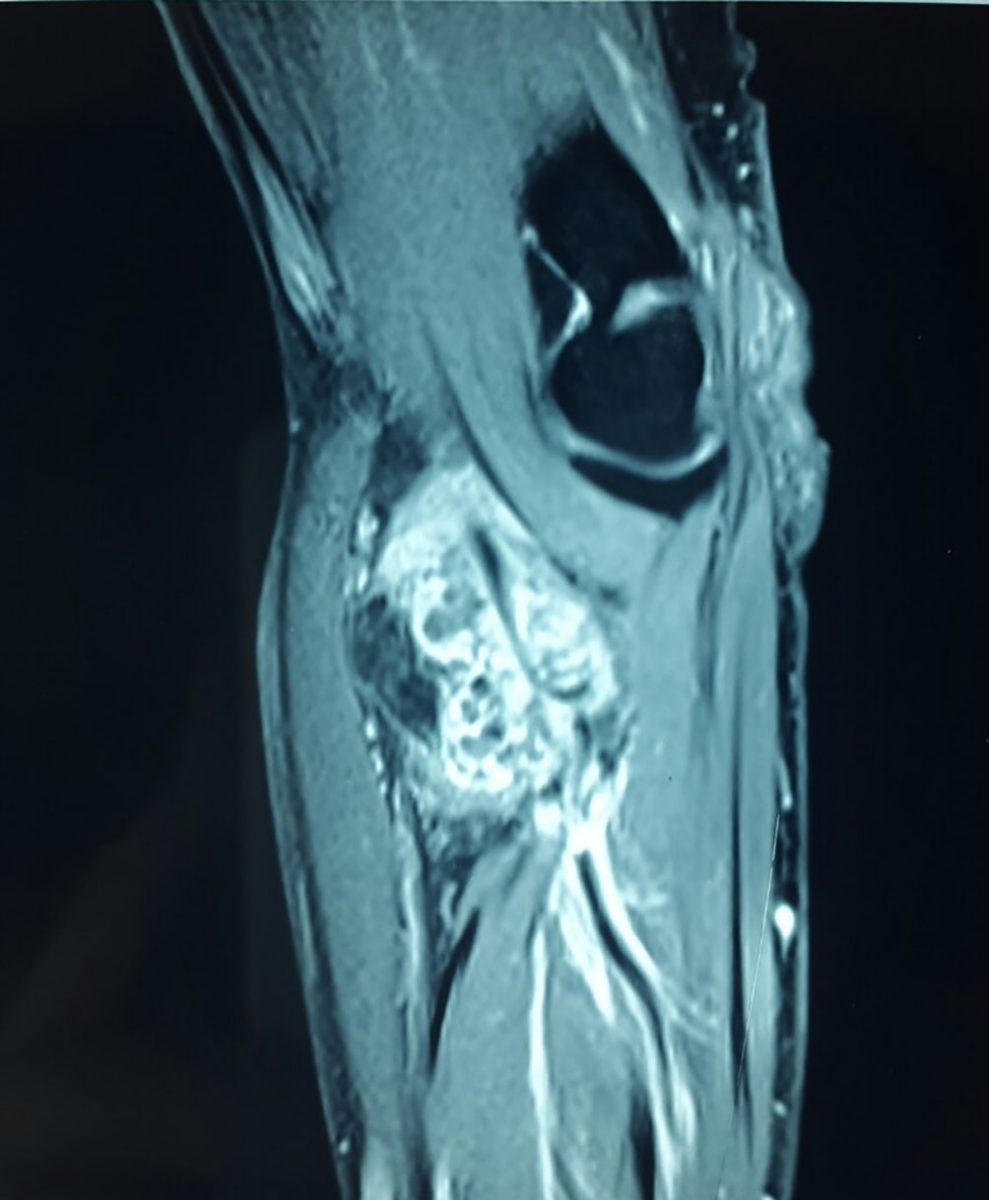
Sagittal magnetic resonance imaging (MRI) image of the left front arm with a lobulated complex mass with frond-like appearance extending from the bicipitoradial bursa.
